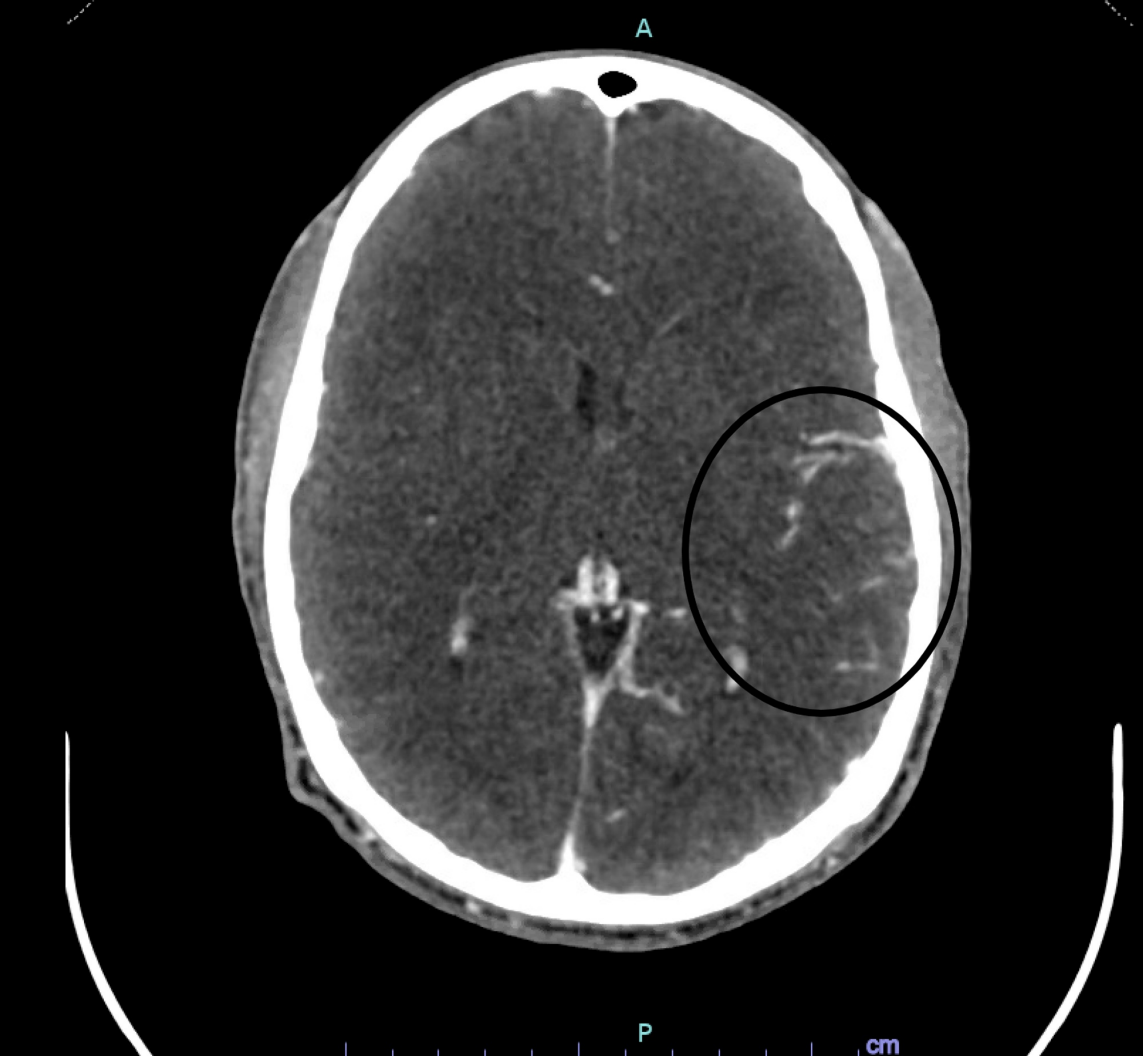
CTA head demonstrating dilation of the distal branches of the left MCA territory
